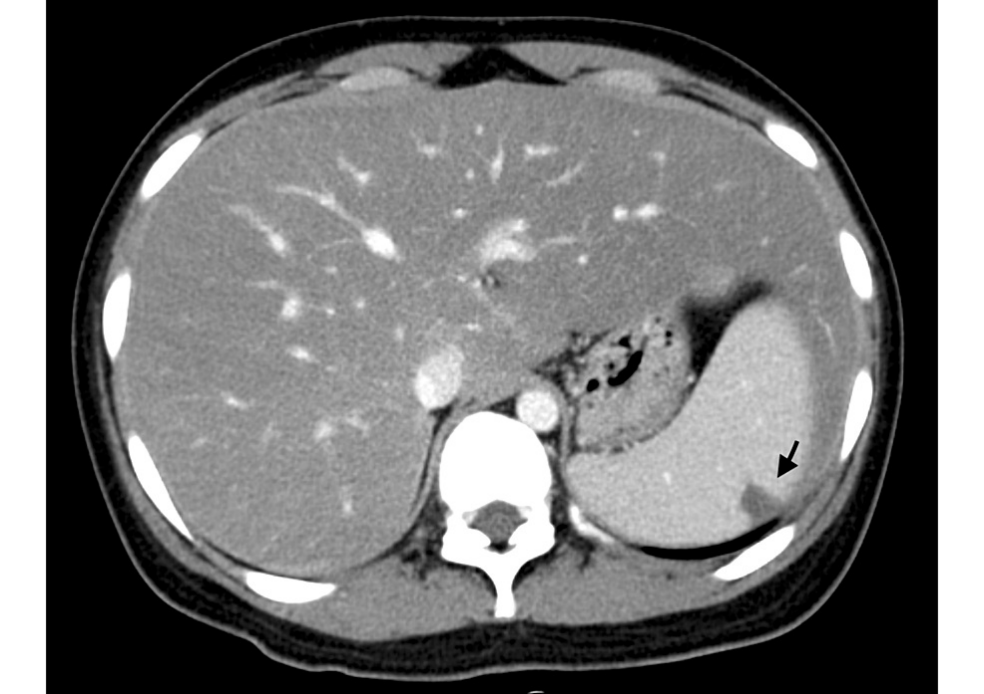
Initial imaging study of the abdomen An initial enhanced CT image of the abdomen shows hepatosplenomegaly and a splenic infarction (arrow).
